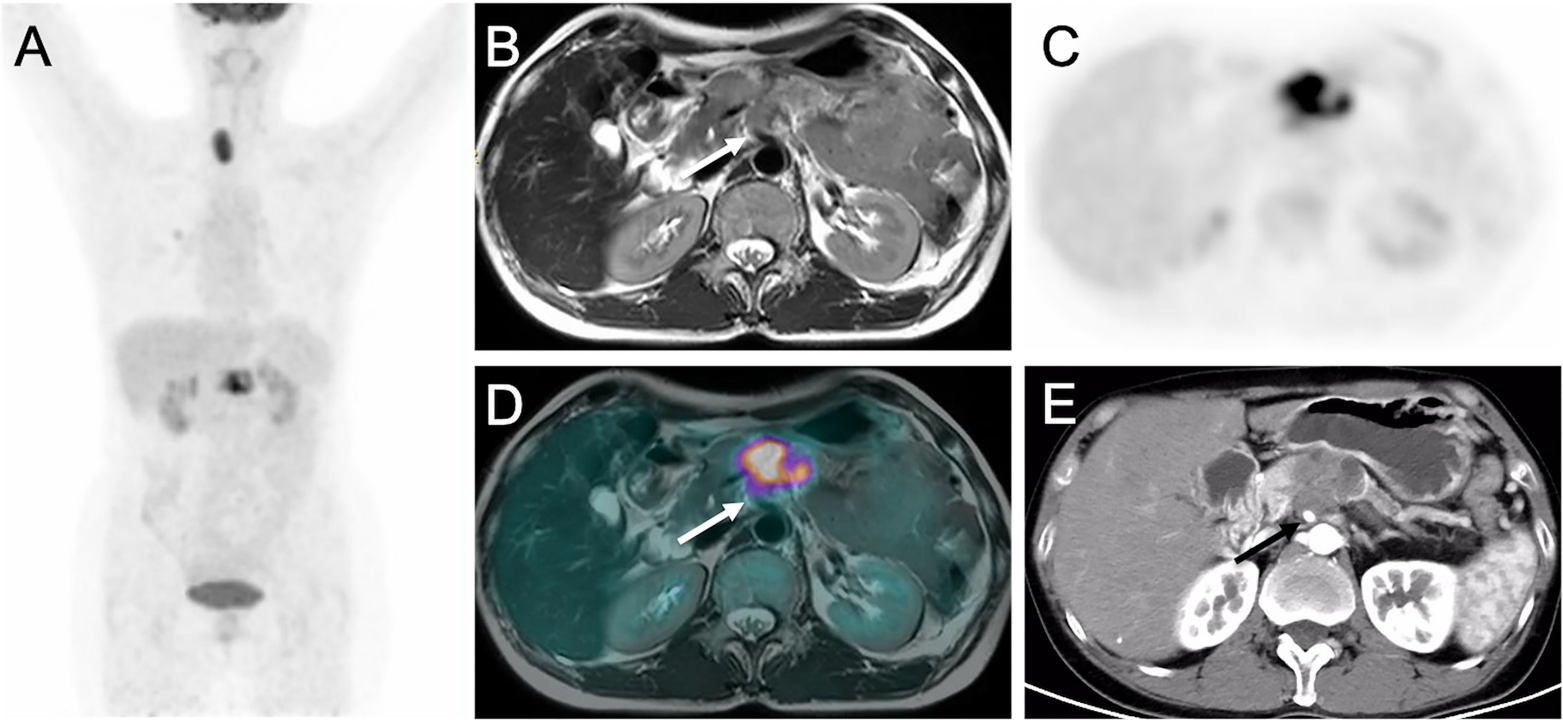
Images of pancreatic ductal adenocarcinoma in 52-year-old woman with vascular invasion. (A) MIP from PET/CT showing increased uptake in pancreas. (B) T2WI, (C) Delayed PET image, (D) Corresponding PET/MR imaging fusion image and (E) Arterial phase CT image show the mass in the body of pancreas encasing superior mesenteric artery (arrows).
